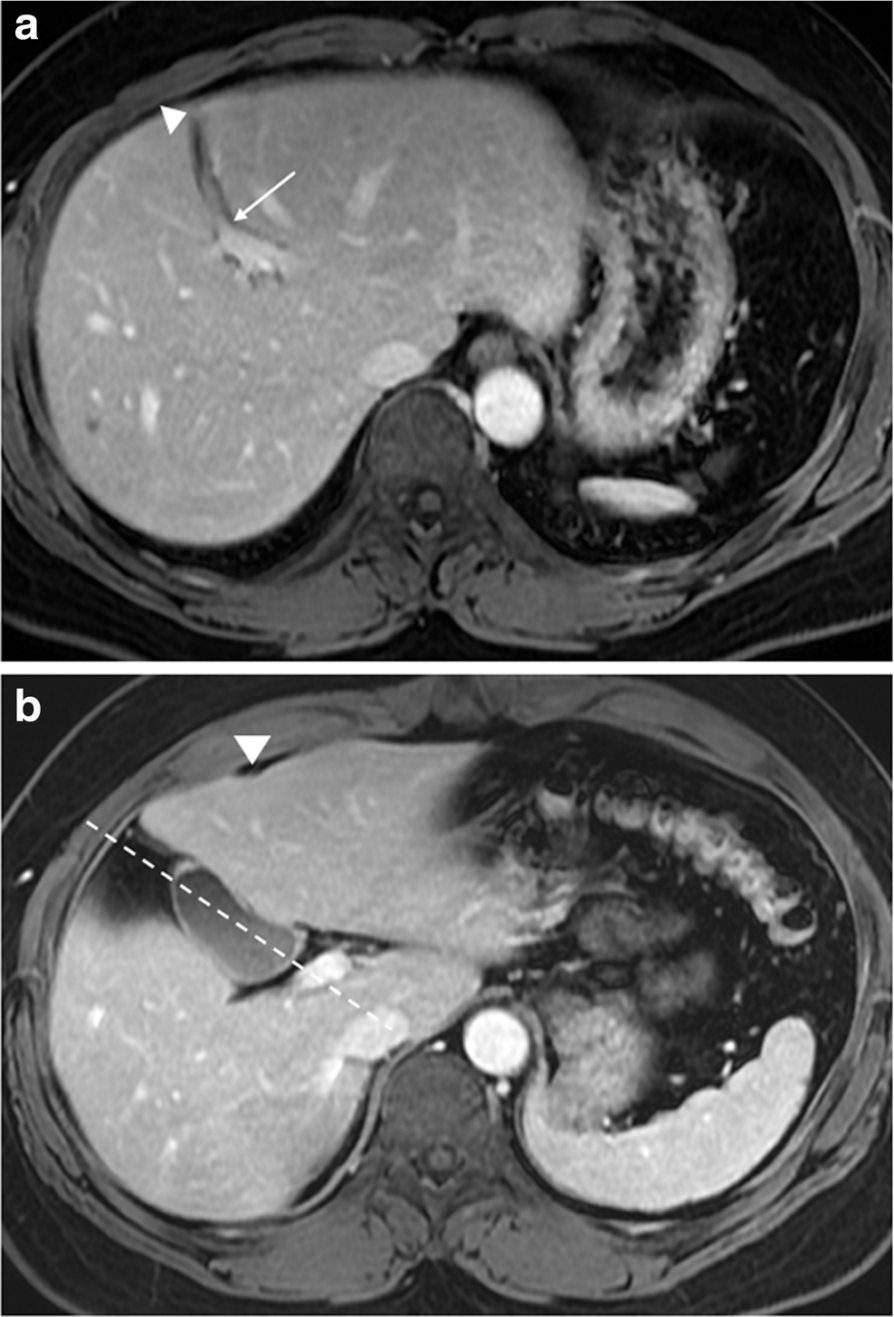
a Contrast-enhanced T1 fat-sat MRI revealing the right paramedian portal vein forming the umbilical portion (*arrow*) of the portal vein and joining with the RSLT (*arrowhead*). b The gallbladder has a normal cholecystic axis (*dotted line*) to the right of the umbilical fissure (*arrowhead*)
